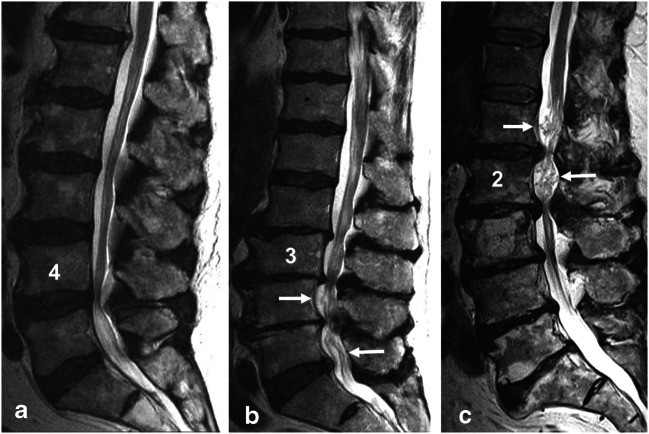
Sagittal T2-weighted images of the lumbar spine showing cauda equina nerve roots: a with no evidence of RNRs since the morphology of the cauda nerve roots is not affected by the stenosis at level L4/L5; b with serpentine-shaped RNRs (white arrows) caudally to the stenosis at level L3/L4 and c with loop-shaped RNRs (white arrows) cranially to the stenosis at level L2/L3
